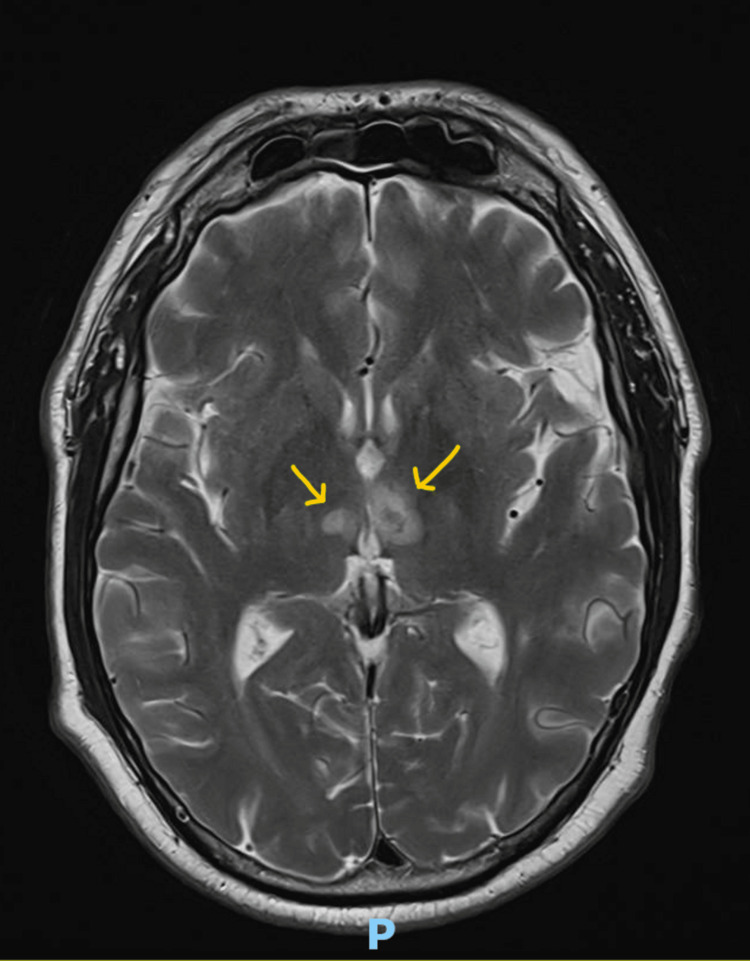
T2-weighted axial MRI imaging of the patient. The gold arrows demonstrate hyperintense signals in the anteromedial thalamic regions bilaterally, suggestive of infarction. These thalamic infarctions form the classical *V sign* associated with AOP syndrome [7]; however, the V shape is less appreciable in this case. AOP, Artery of Percheron
